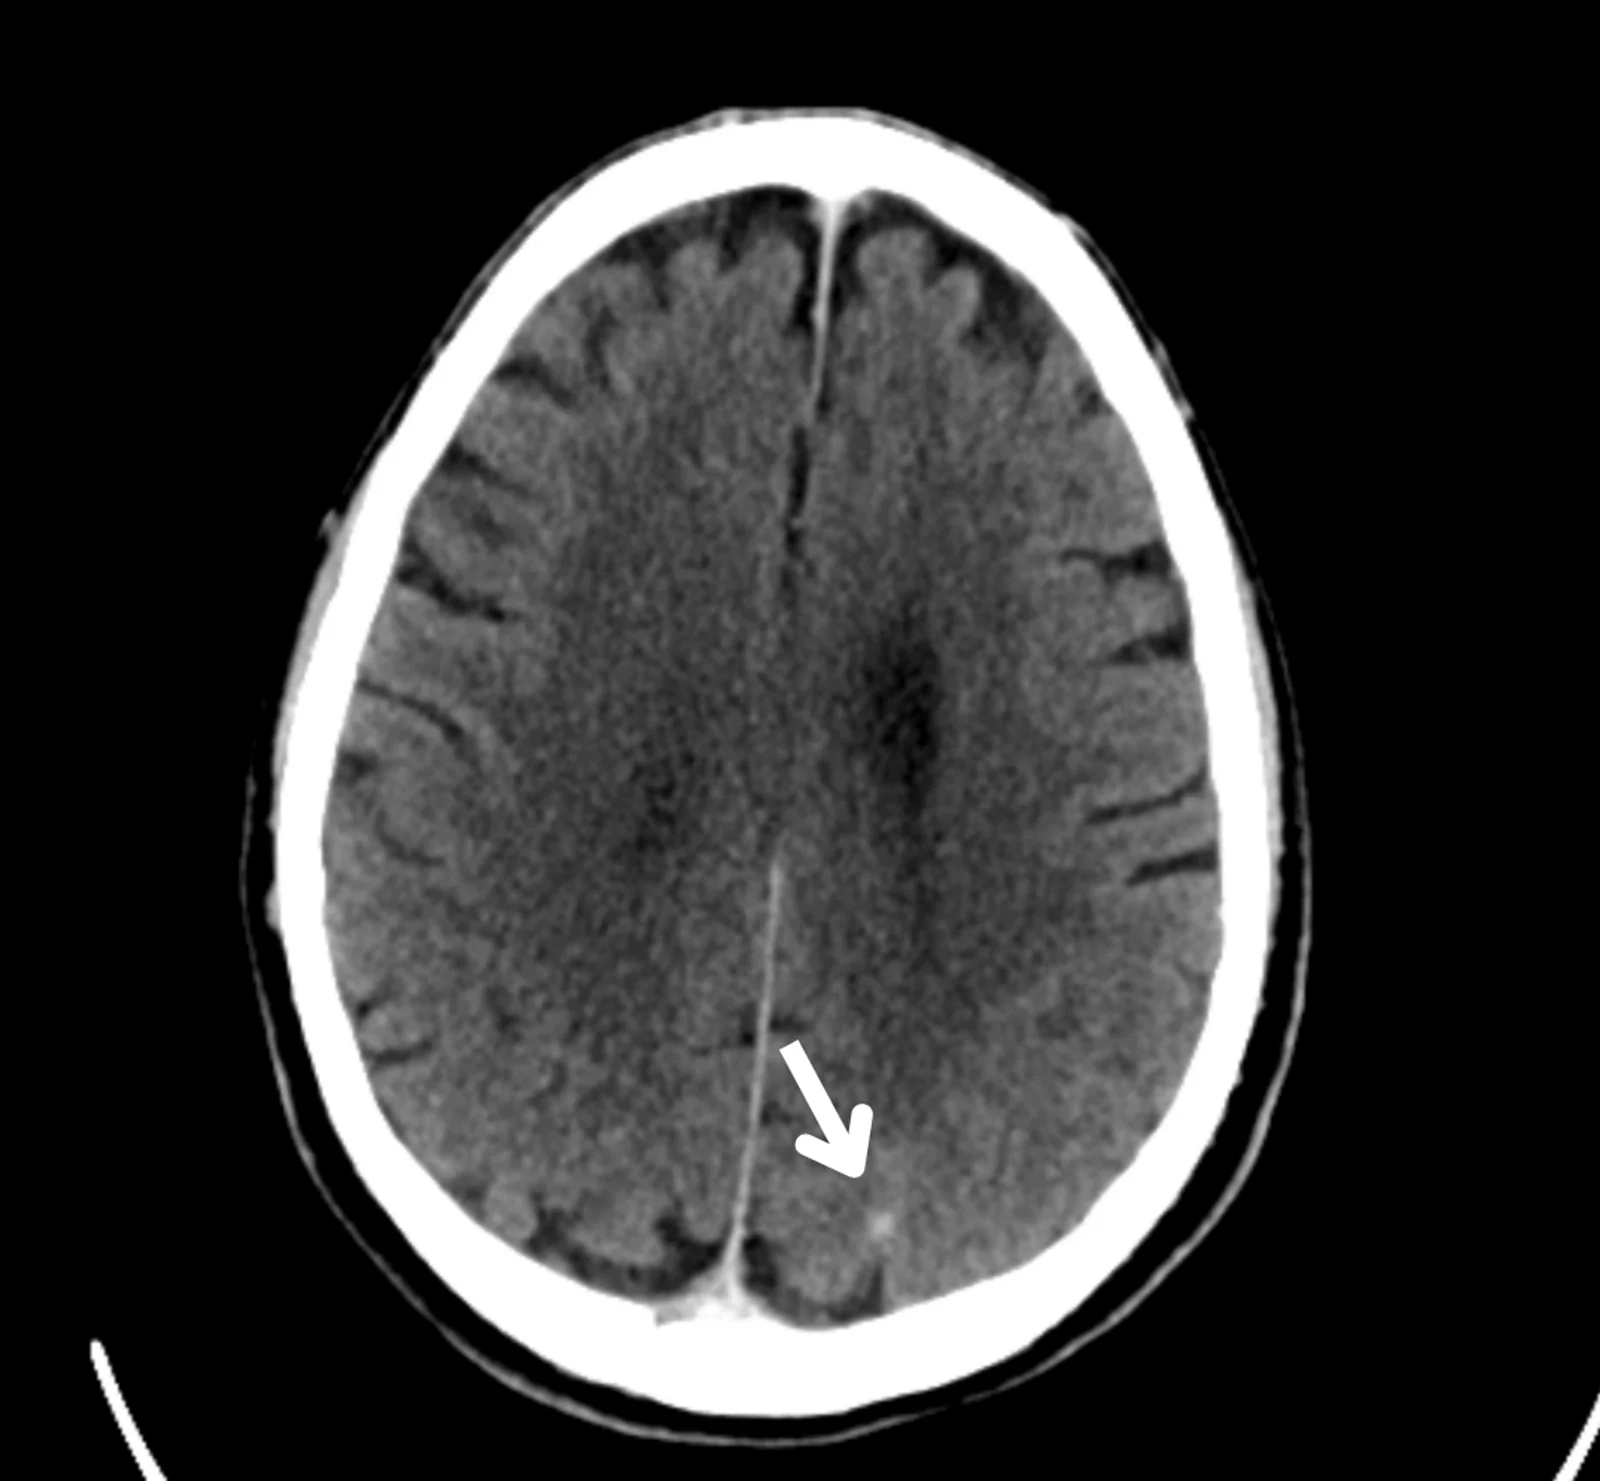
Computed tomography (CT) head with contrast Arrow showing a subtle focal hyperdensity along the left parietal extra-axial space, concerning for intracranial hemorrhage, which prompted further neurovascular evaluation
